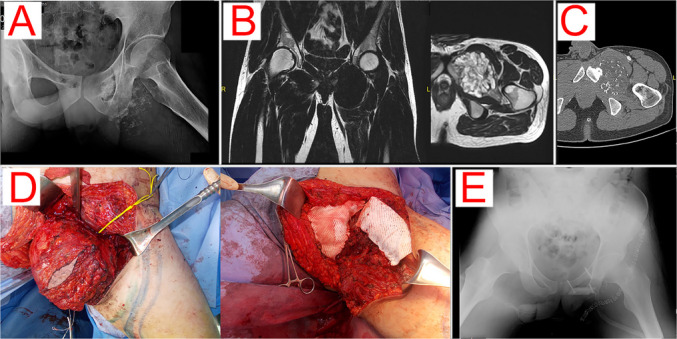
Twenty-six-year-old male patient with Grade 1 CS of the obturator foramen. A) Preoperative X-ray revealing calcifications within the matrix; B) coronal T1-weighted MRI on the left showing a large hypointense mass; axial T2-weighted MRI on the right displaying hyperintense signal; C) CT scan clearly depicting calcifications within the matrix; D) intraoperative photos of the large inguinalmass (biopsy scar in the foreground) on the left and reconstruction using homoplastic bone reinforcement and Gore-Tex membrane on the right; E) postoperative X-ray
